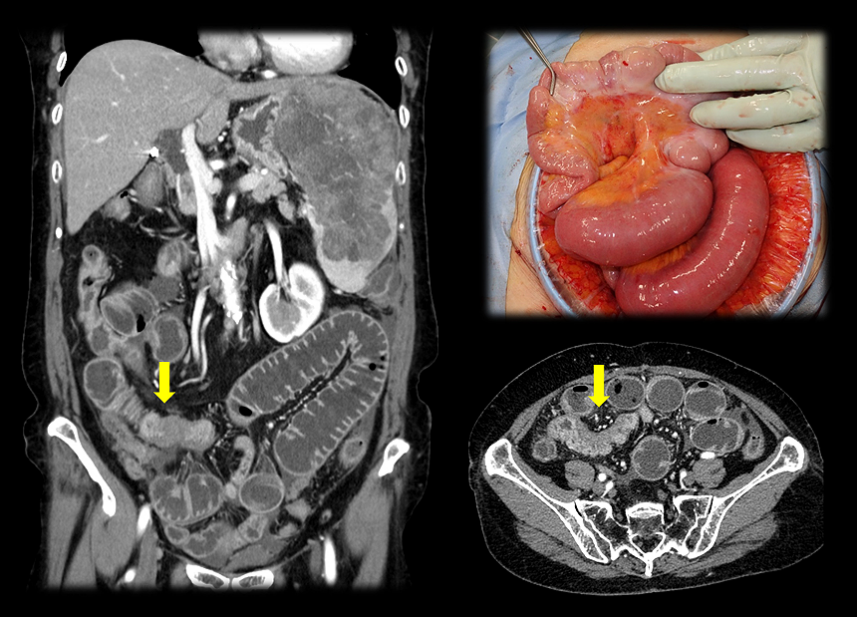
Computed tomography revealed small bowel wall thickening (arrow), which caused a mechanical bowel obstruction. At surgery, peritoneal dissemination and ascites were confirmed. The patient underwent a partial resection of the intestine.
